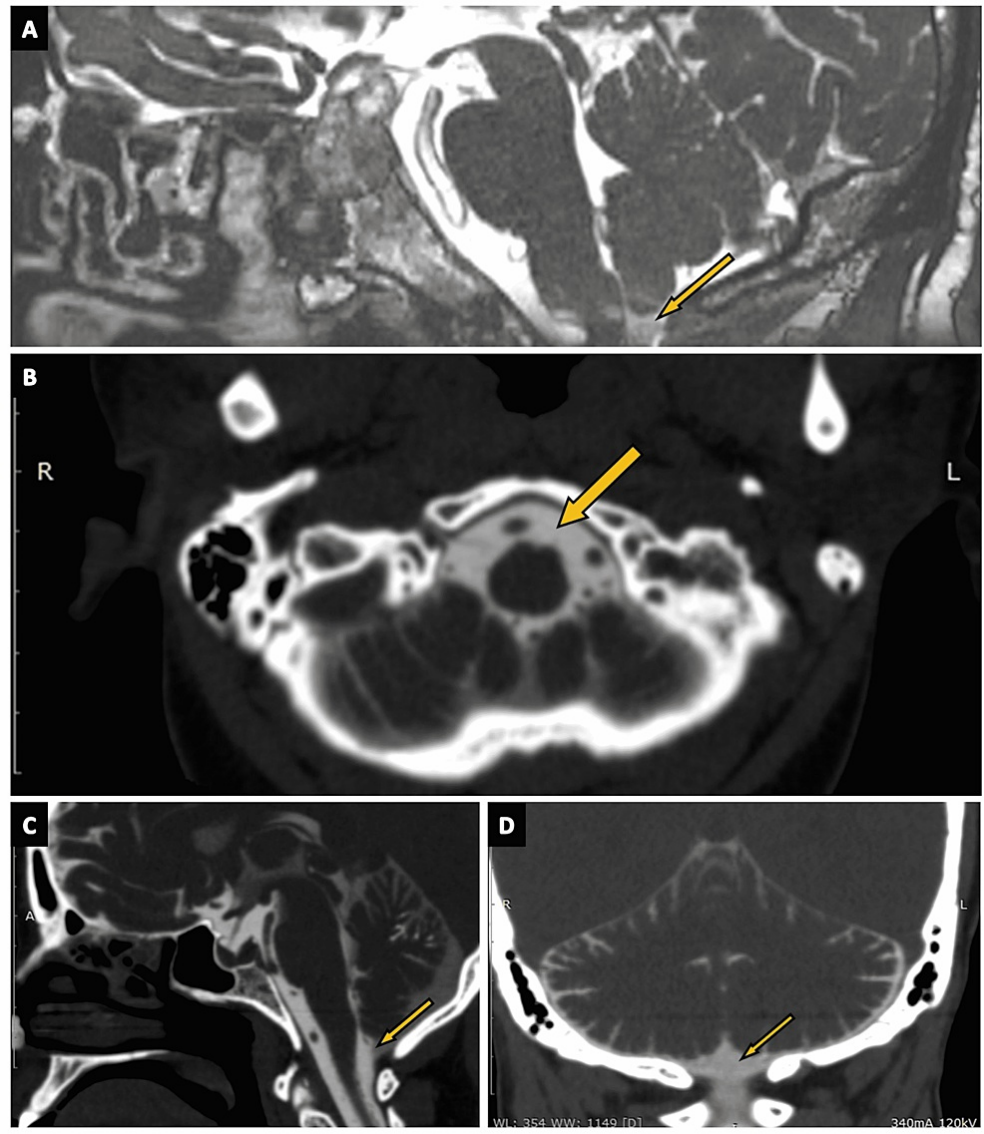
The cisterna magna. (A) The cisterna magna (arrow) in the sagittal plane of the three-dimensional (3D) constructive interference in steady state (CISS) MRI sequence. (B), (C), and (D) show the cisterna magna (arrows) as seen in the axial, sagittal, and coronal planes of CT cisternography, respectively, in a case of CSF rhinorrhea.
